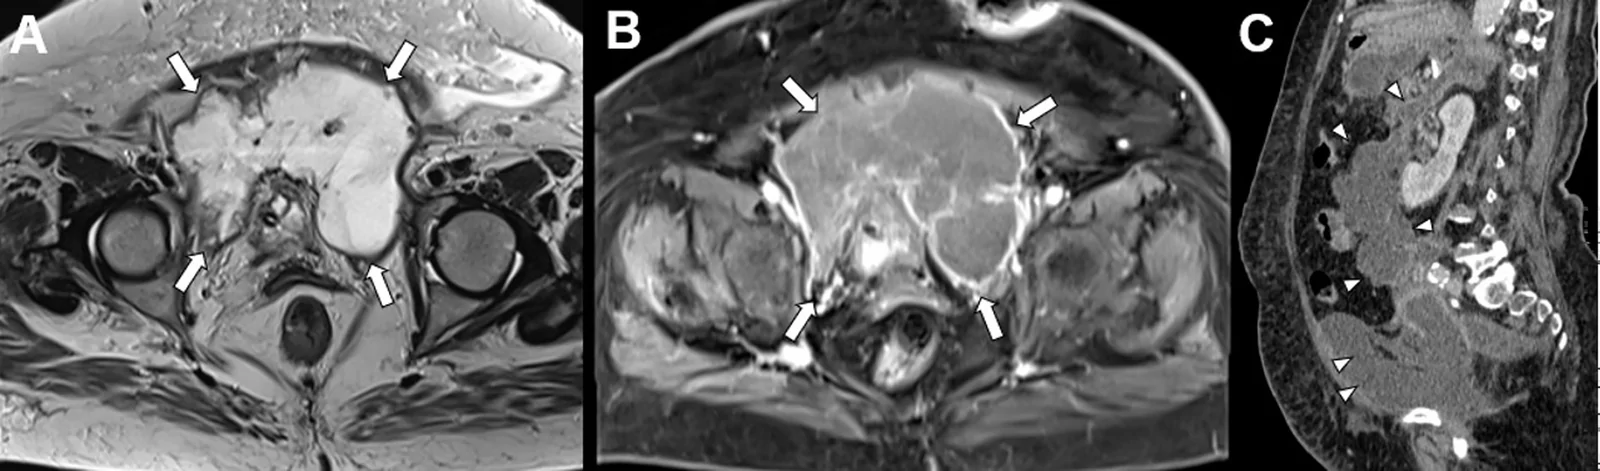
Necrotizing pancreatitis–related fluid collection extending into the space of Retzius. Axial T2-weighted (A) and post-contrast T1-weighted (B) MR images demonstrate a peripherally enhancing, multiloculated fluid collection in the space of Retzius extending into the paravesical spaces, producing the “molar tooth” sign (white arrows). A sagittal contrast-enhanced CT image (C) demonstrates continuity of the peripancreatic fluid collection into the retroperitoneum, as well as the space of Retzius (white arrowheads)
